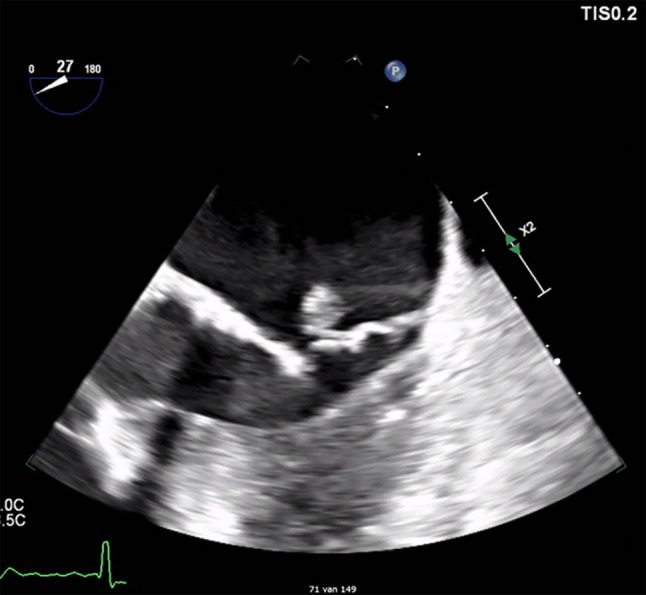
Transoesophageal echocardiographic image of mitral valve with vegetation on posterior valve leaflet
